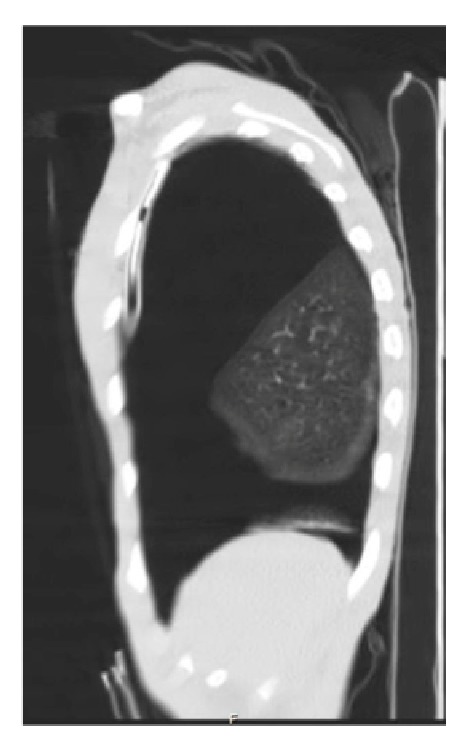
Chest CT scan sagittal cuts showing the chest tube location.
